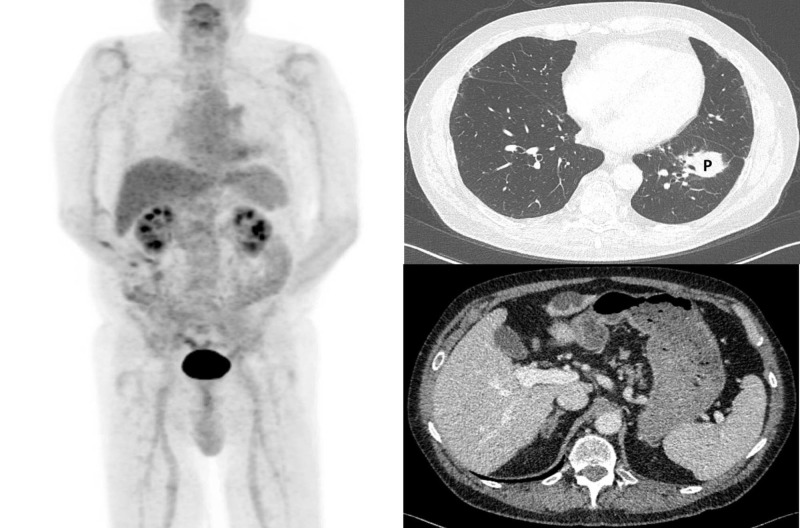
Surveillance imaging after completion of nivolumab and left lung SBRT at 30 months from diagnosis. PET/CT maximum intensity projection (left), and representative transverse CT chest (right, top) and CT abdomen (right, bottom) images are shown. There is no evidence of metabolically active disease. The left lung parenchymal lesions treated with SBRT are stable in size and morphology (P; right, top). The initially seen right-sided pulmonary parenchymal nodules have demonstrated stable partial response. The omental mass has radiographically resolved (right, bottom). PET/CT: Positron emission tomography/computed tomography; SBRT: Stereotactic body radiation therapy.
